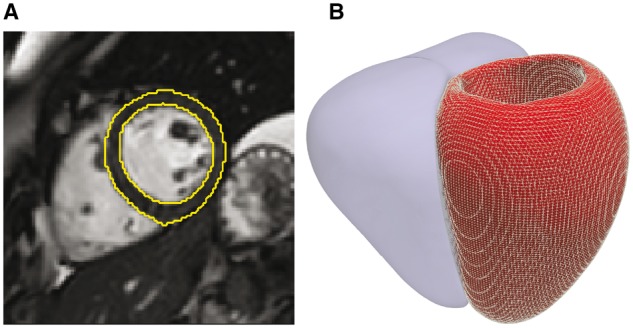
Computational image analysis. (A) Short axis cardiac magnetic resonance image demonstrating automated segmentation of the endocardial and epicardial boundaries of the left ventricle. (B) The segmentation is used to construct a three dimensional mesh of the cardiac surfaces (left ventricle shown as a mesh, right ventricle shown as a solid) that is co-registered to a standard coordinate space. Phenotypic parameters, such as wall thickness, are then derived for each vertex in the model
